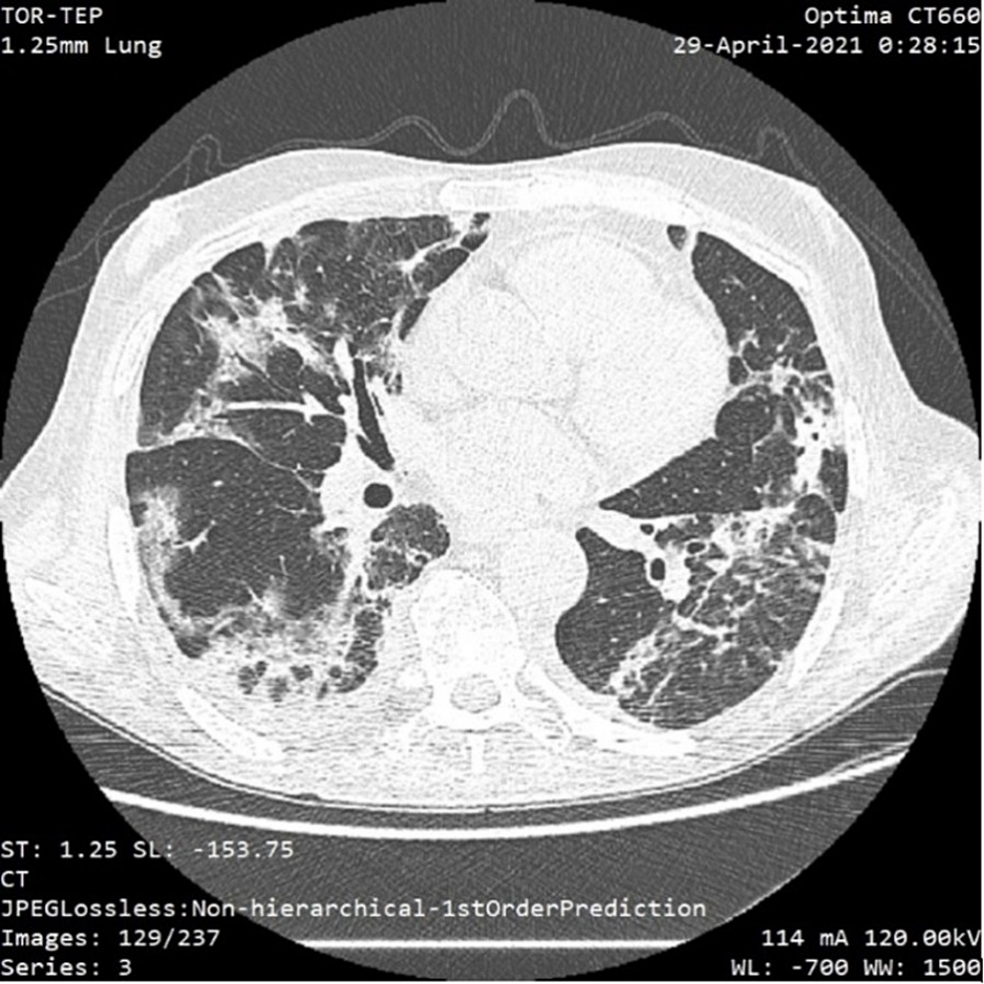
CT scan showing minimal bilateral pleural effusions and typical glass opacities and fibrosis in approximately 35% of the lung parenchyma
